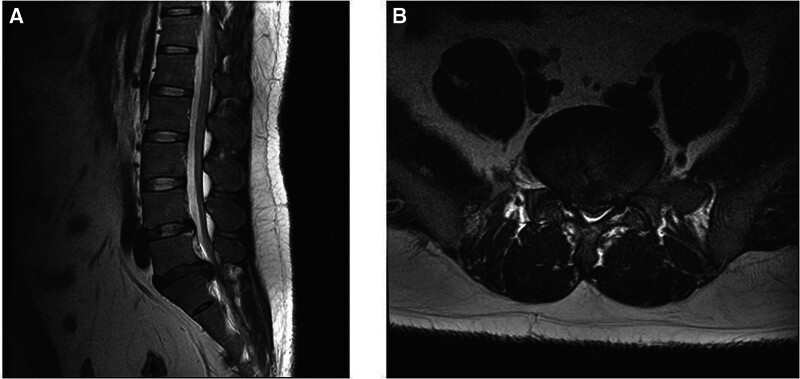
(August 05, 2011) L-spine magnetic resonance imaging. (A) T2 sagittal view; (B) T2 axial view.
